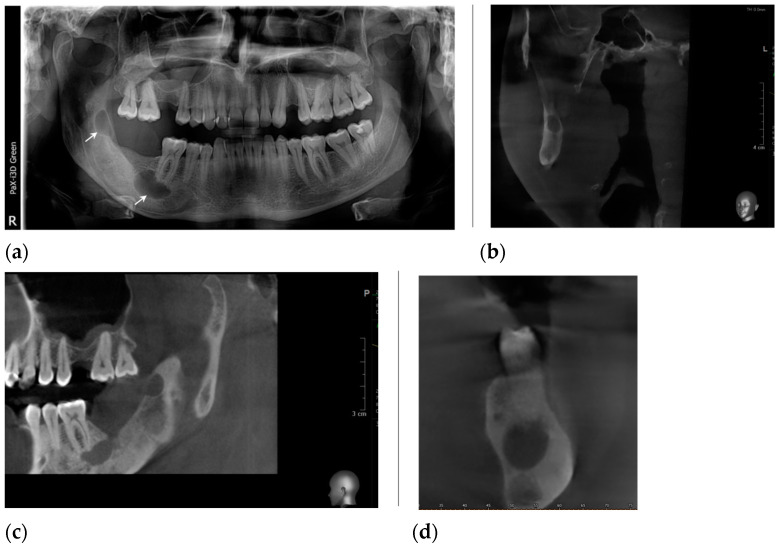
The radiological aspects: (a) panoramic radiograph demonstrate two well-defined osteolytic lesions: one at the apical region of tooth #46 (arrow), without a mandibular canal involved, and another at the anterior margin of the ascendant ramus (arrow); (b) the CBCT, coronal view of the affected ascending ramus; (c) the CBCT, coronal view highlights the panoramic radiograph findings; (d) the CBCT, sagittal view demonstrates the lesion contact with the molar #46.
